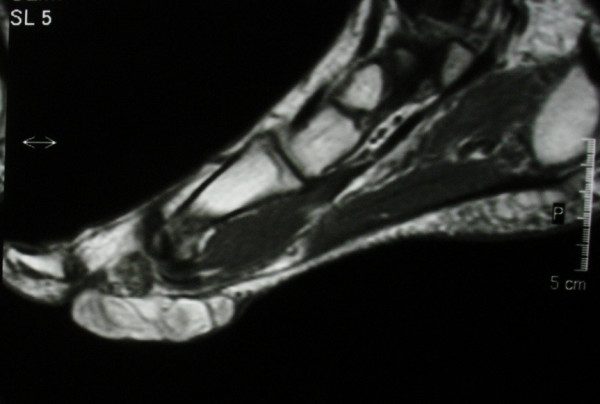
The corresponding sagittal T1-weighted contrast enhanced image. The non-fatty component does not show any apparent enhancement.
